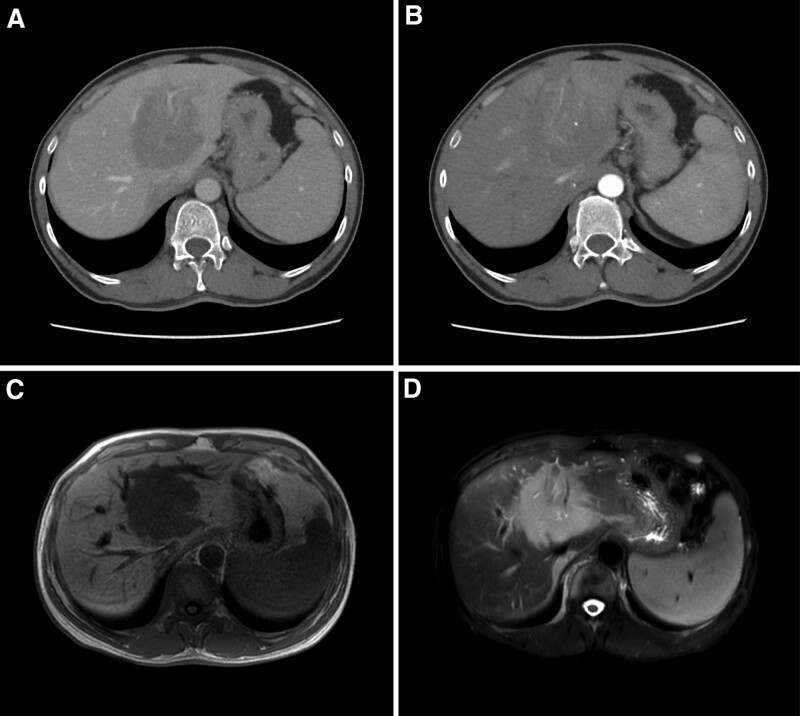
(A) Hepatic enhanced CT venous phase, in this image, a mass-like heterogeneous low-density shadow with indistinct borders is observed in the hepatic hilum region. (B) Hepatic enhanced CT arterial phase, the arterial phase CT scan reveals an irregular enhancement pattern within the liver. During the delayed phase, the degree of enhancement decreases. (C) Hepatobiliary pancreatic magnetic resonance cholangiopancreatography (MRCP) T1 phase, The T1 phase MRCP image exhibits a uniform low signal intensity throughout the liver. (D) Hepatobiliary pancreatic MRCP T2 phase, in the T2 phase MRCP image, a uniform high signal intensity is observed within the liver. CT = computed tomography.
